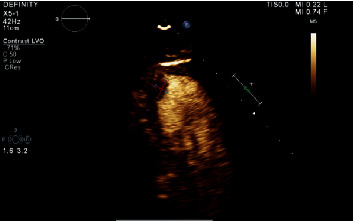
Transthoracic echocardiogram with left ventricular thrombus.
